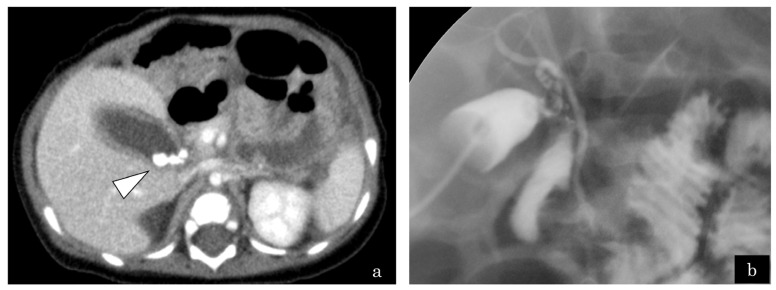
Case 6: A 4-month-old girl presented with vomiting and revealed gallbladder stones on ultrasonography. (a) Enhanced computed tomography revealed multiple small gallstones and gallbladder swelling (white arrowhead). (b) The patient developed septic shock due to cholangitis. Emergency gallbladder drainage was performed. No communication between bile and pancreatic duct was observed by cholangiography.
